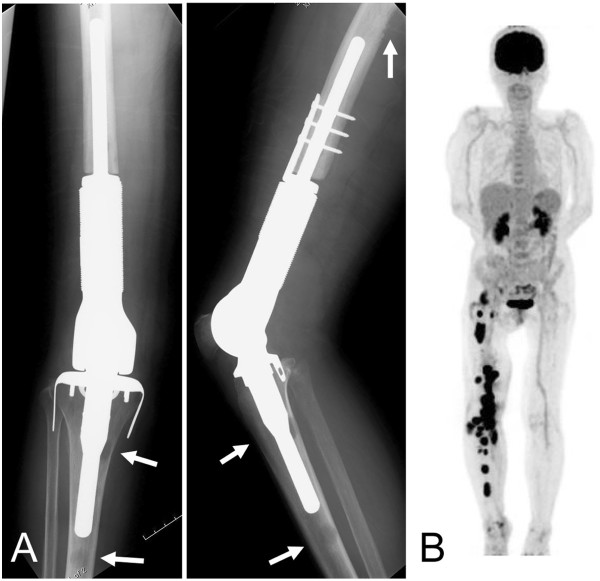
Postoperative epithelioid angiosarcoma images. (A) Three months after tumor resection and artificial joint replacement, plain radiographs show multiple osteolytic lesions at the femur and the tibia (arrows). (B) [^18^F]-2-fluoro-2-deoxy-D-glucose positron emission tomography shows multiple lesions extending from the pelvic cavity to the lower extremity.
